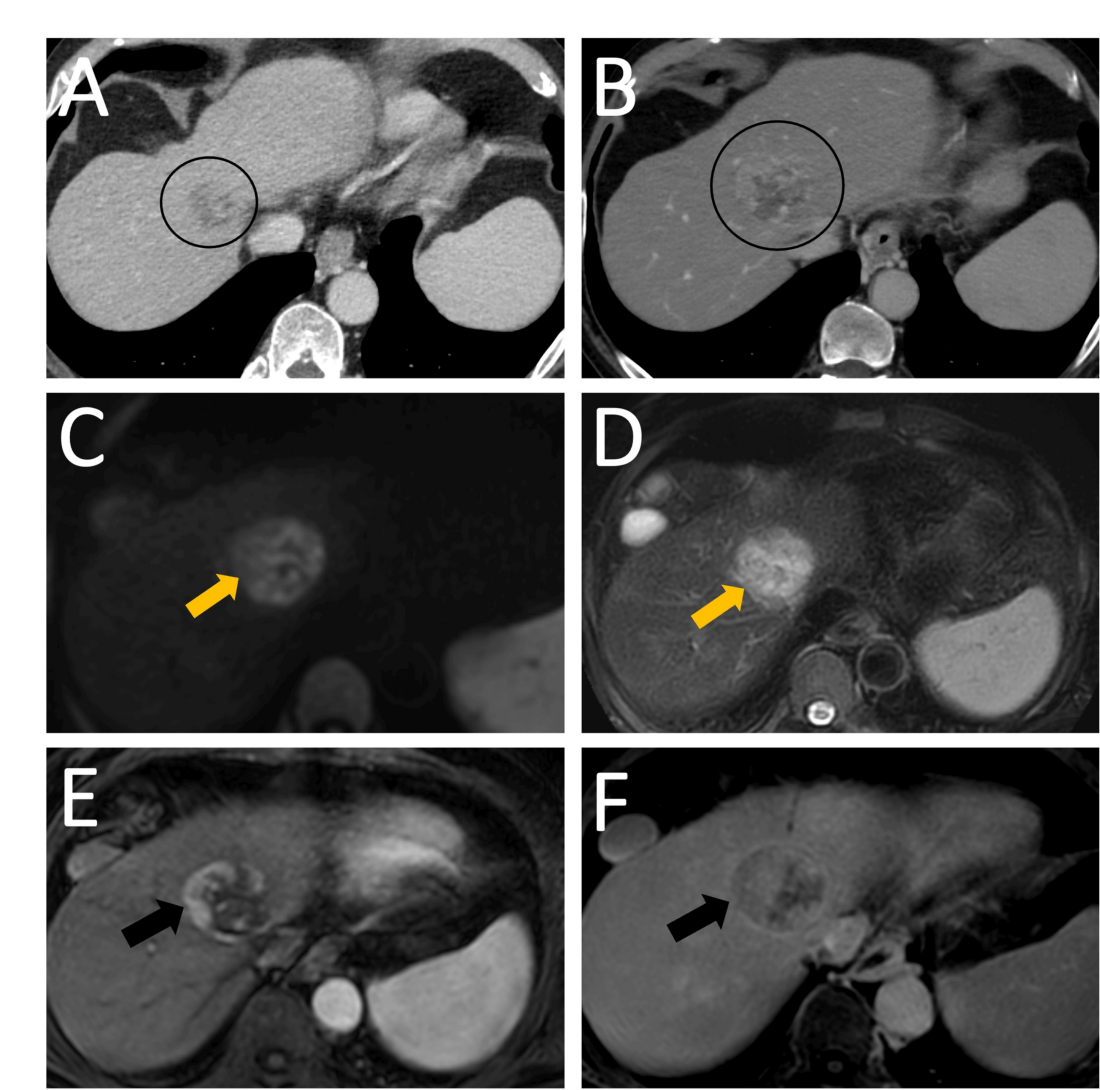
A) 6-month follow-up post-contrast axial CT scan with a decrease in the size of the hepatic lymphoma (black circle in A). B) 1-year follow-up post-contrast axial CT scan with an increase in the size of the lesion and a new heterogenous enhancement (black circle in B). C) Restricted diffusion on MRI diffusion-weighted imaging within the same lesion (yellow arrow in C). D) Axial T2-weighted MRI with hyperintense lesion compared to the background liver (yellow arrow in D). E) Axial T1-weighted post-contrast MRI in arterial phase with a non-rim hyperenhancement (black arrow in E). F) Axial T1-weighted delayed post-contrast MRI with washout and enhancing capsule (black arrow in F)
